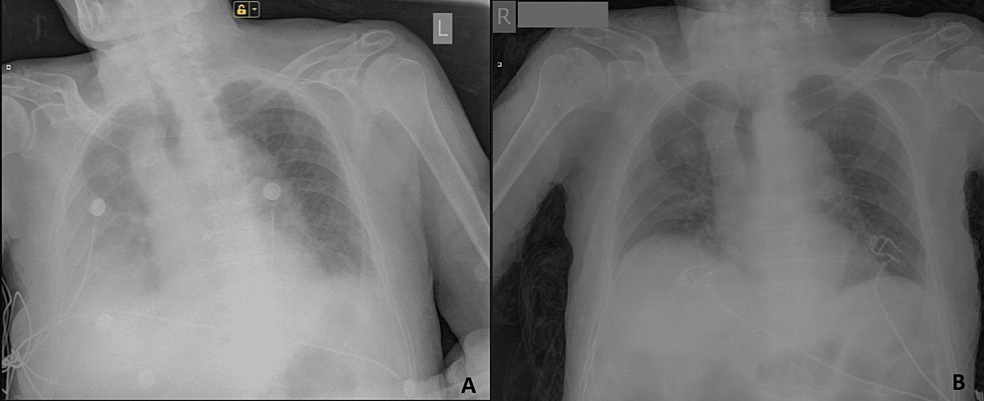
Chest X-ray A: on the day of the COVID-19 diagnosis; B: on the 14th day after treatment with high-dose colchicine was started.
